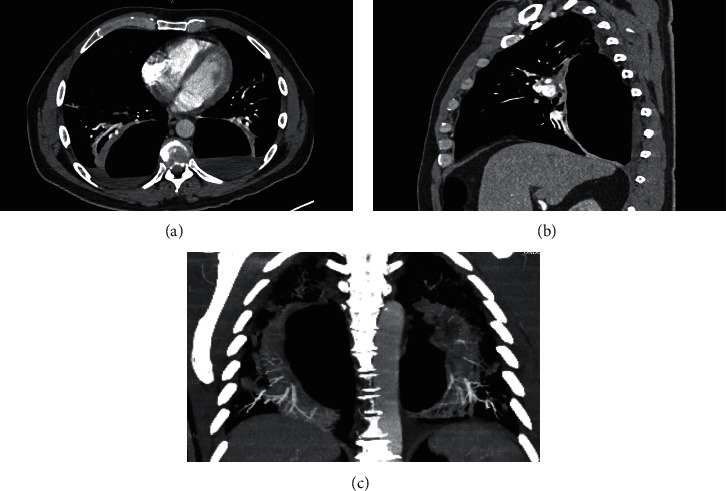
CTA from the outside hospital imaged prior to transfer demonstrating large cystic lesions in the (a) axial, (b) sagittal, and (c) coronal views.
